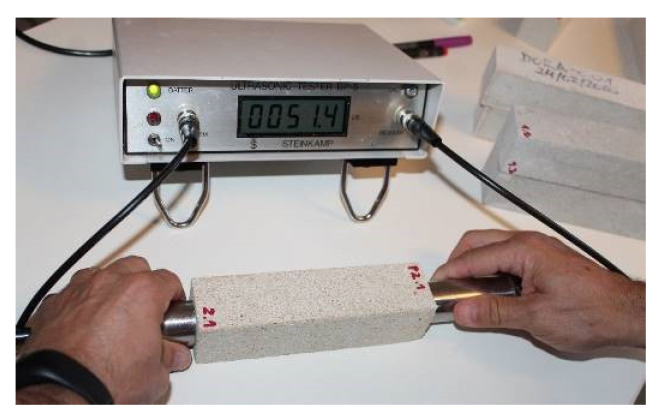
Sample P2.1 (Bateig Beige Hydra) subjected to direct ultrasonic testing.
